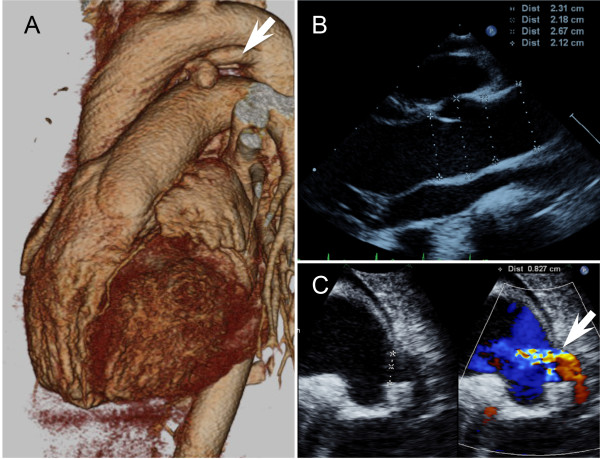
Cardiovascular phenotypes of the present case. Imaging studies from a 3D-reconstructed CT scan (A) and echocardiographies (B and C) are shown. The case had abnormally dilated the aortic root (A, B) and the patent ductus arteriosus (arrows in A and C). Note that, in panel C, the shunt flows are present across the ductus.
